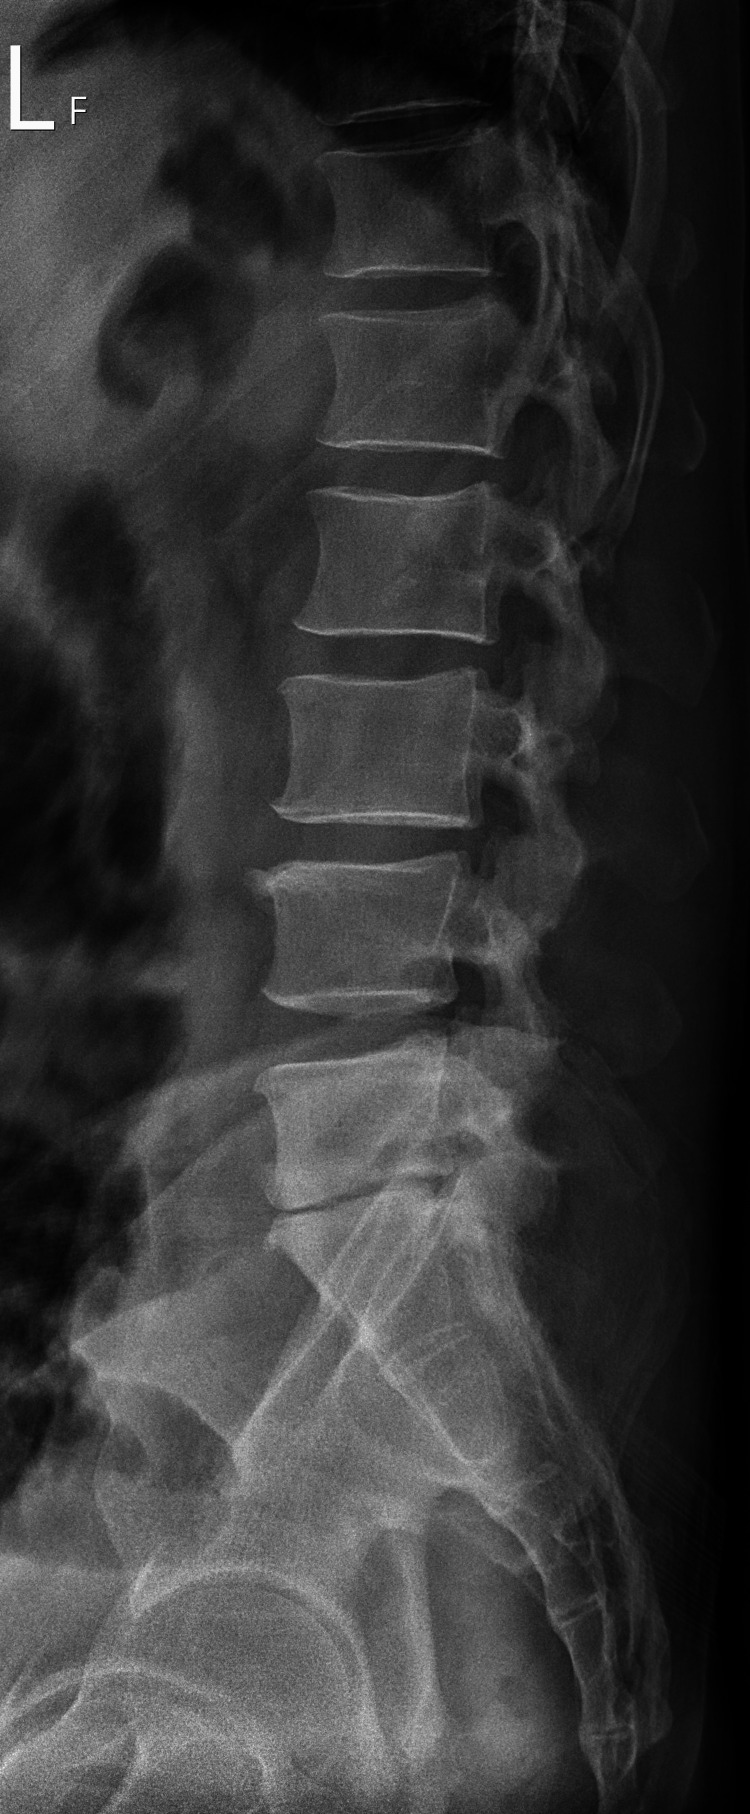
Radiography of the lumbar spine shows narrowing of the disc space at L5/S1 and some anterior marginal osteophytes.
